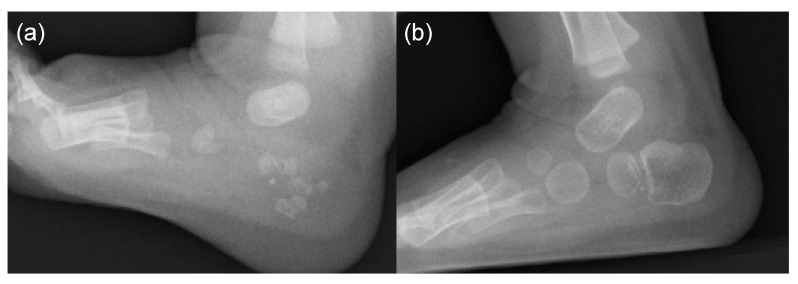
Lateral foot radiographs of a FOP boy at 3 months of age (a) and 11 months of age (b). Punctate multiple ossifications in early infancy gradually changed to double ossification centers.
